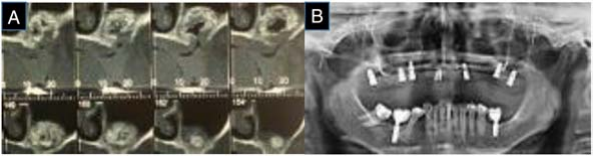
a CT slices (oroantral communication). b Panoramic radiograph (follow-up)
